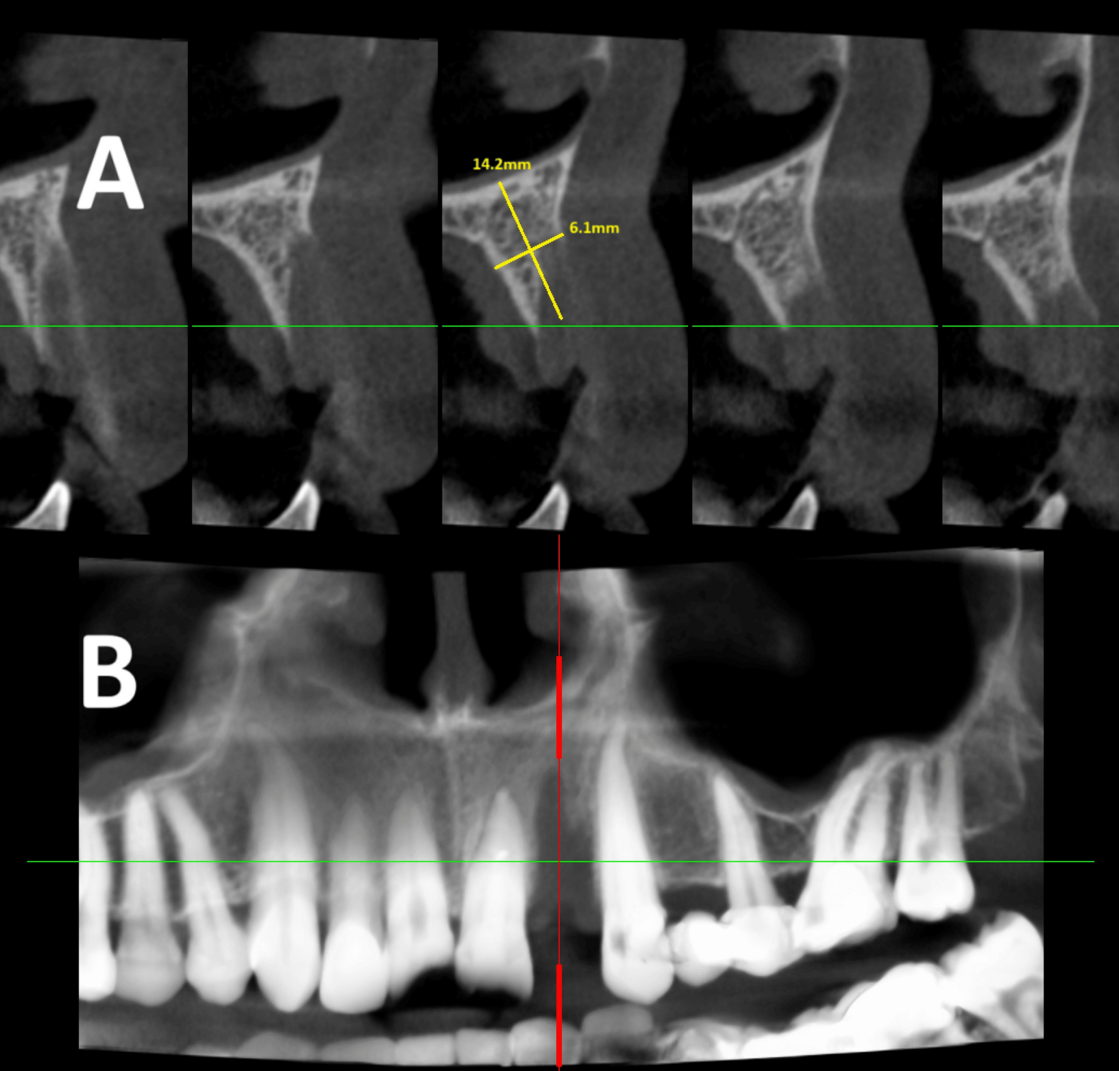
(A) Sagittal and (B) coronal views of CBCT images before implant placement Bone volume measurements are highlighted in yellow. CBCT, cone beam CT
